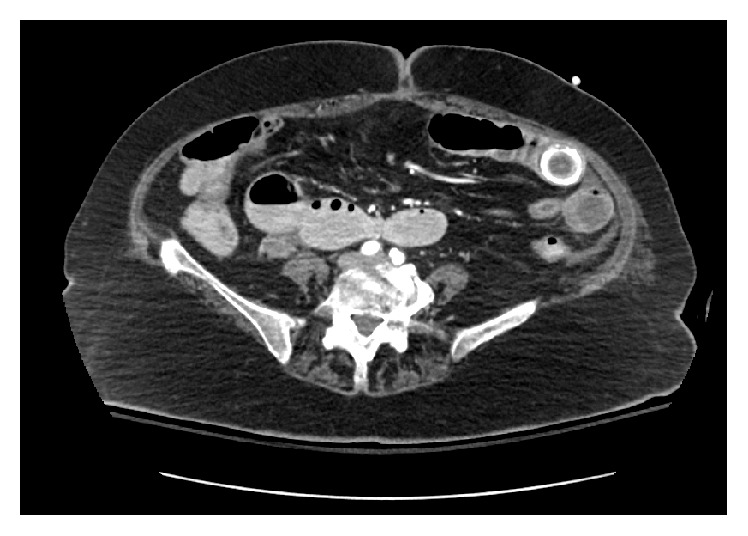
Abdomen and pelvis CT revealing intraluminal gallstone in the left lower quadrant leading to high grade bowel obstruction.
